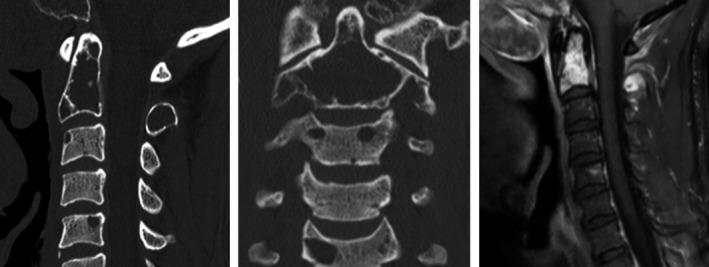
Images of C2 lesion MRI T1 images showed multiple bone lytic lesions. The largest at level of C2 involves the entire C2 dens and bilateral pedicles showing T1 hypointensity, and STIR hyperintensity.
